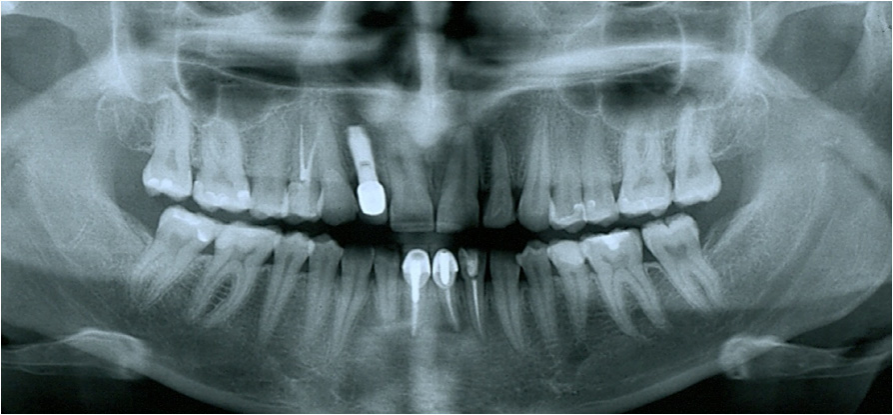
Panoramic radiograph of the same patient 5 years after implant insertion.
